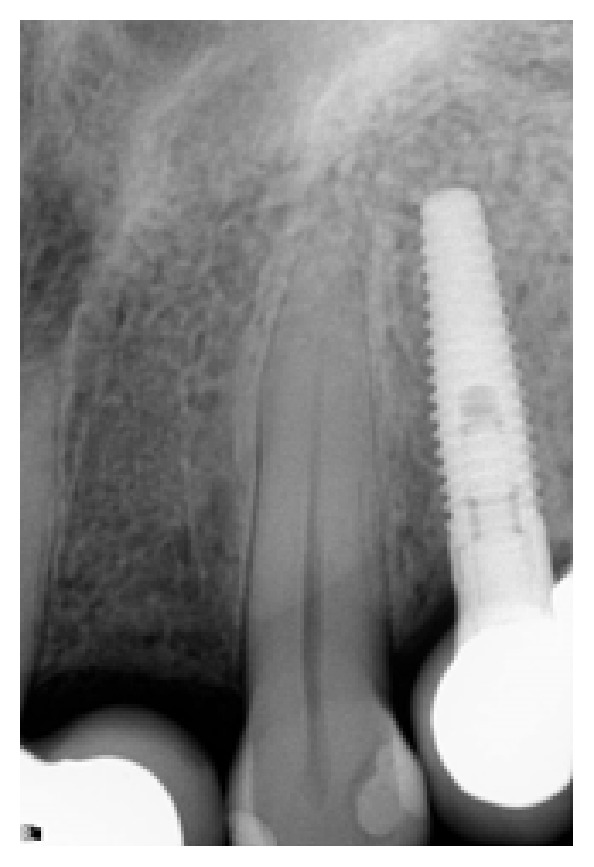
Periapical radiograph of the maxillary anterior region.
